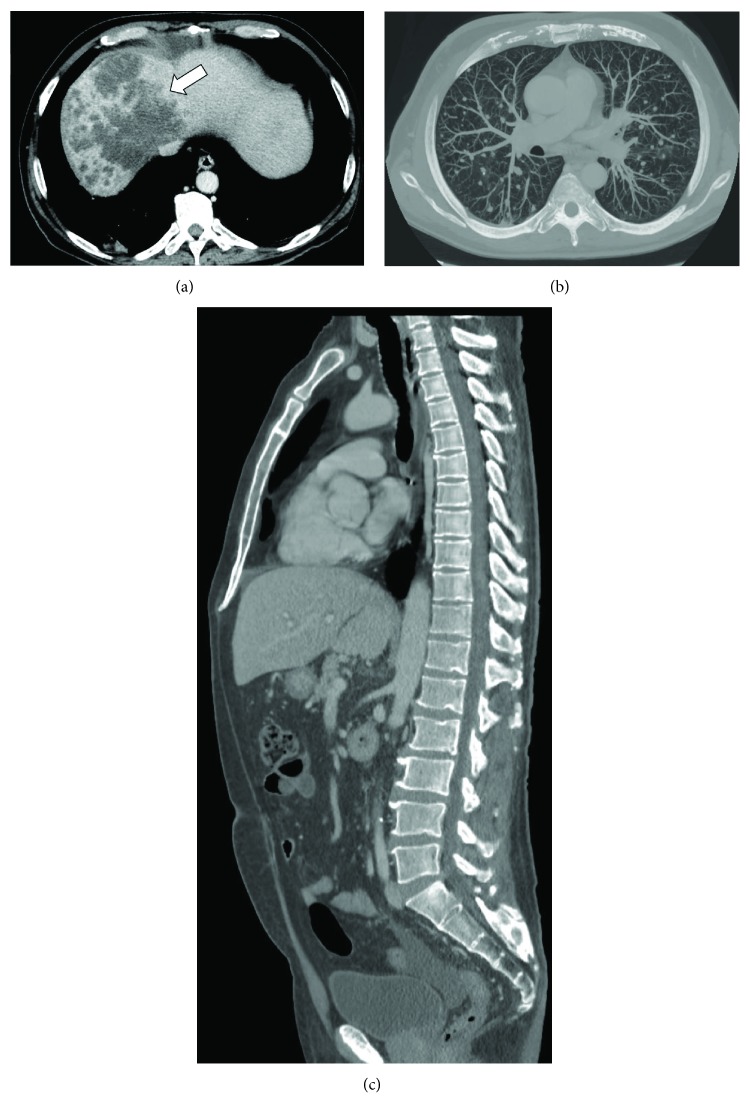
(a) Portal phase CT scan shows large, hypoattenuating mass (arrows) in the right hepatic lobe, consistent with mass-forming peripheral cholangiocarcinoma (arrow). (b) Maximum intensity projection reconstruction of axial CT scan of the chest demonstrates multiple bilateral lung nodules consistent with metastasis. (c) Sagittal CT reconstruction of the spine showed no bony lesions.
